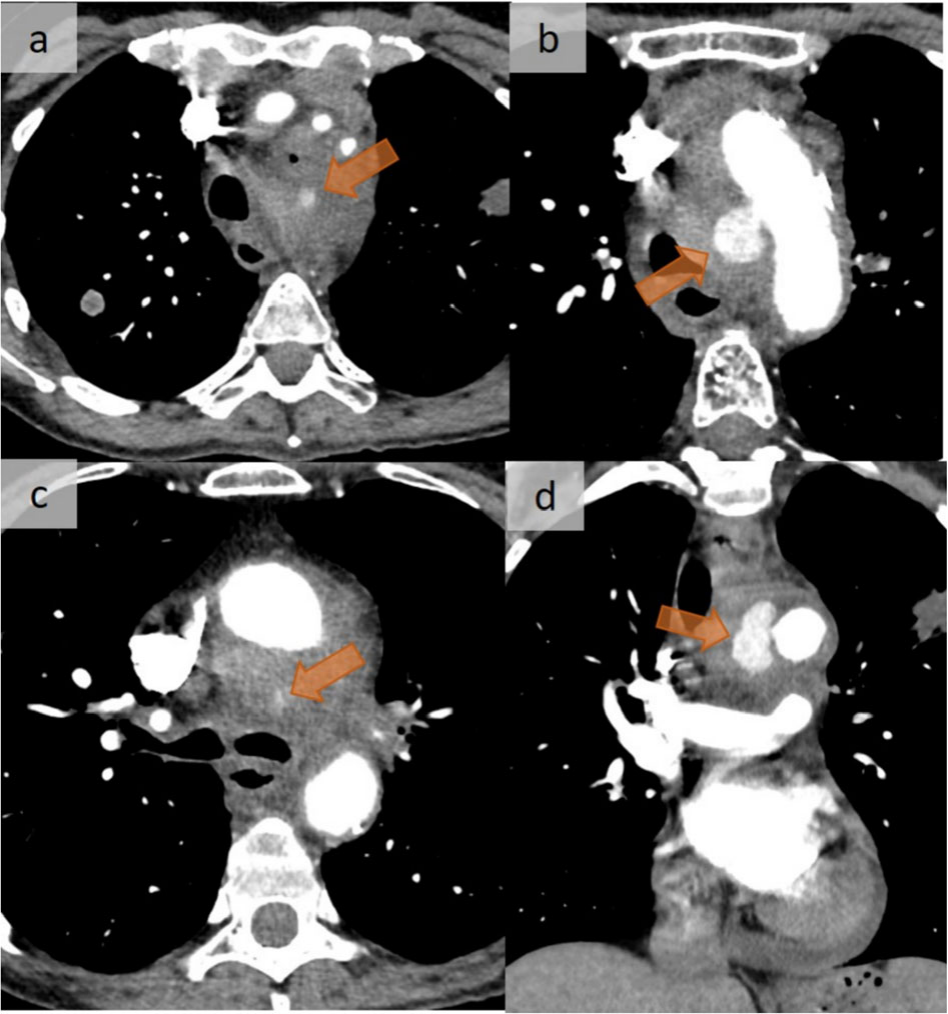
Post-mediastinitis aneurysm. Fatal, false aneurysm complicating a mediastinitis following an EBUS procedure. EBUS had been performed in the context of a suspicion of stage IV lung cancer. Contrast-enhanced CT was performed 35 days after EBUS because the patient complained of chest pain. Successive axial CT images (a–c) show a diffuse infiltration of the mediastinal fat with a false aneurysm (arrow) that developed on the right side of the aortic arch. Coronal reformation shows the cranio-caudal extent of the false aneurysm (arrow) (d). A pericardial effusion is present
